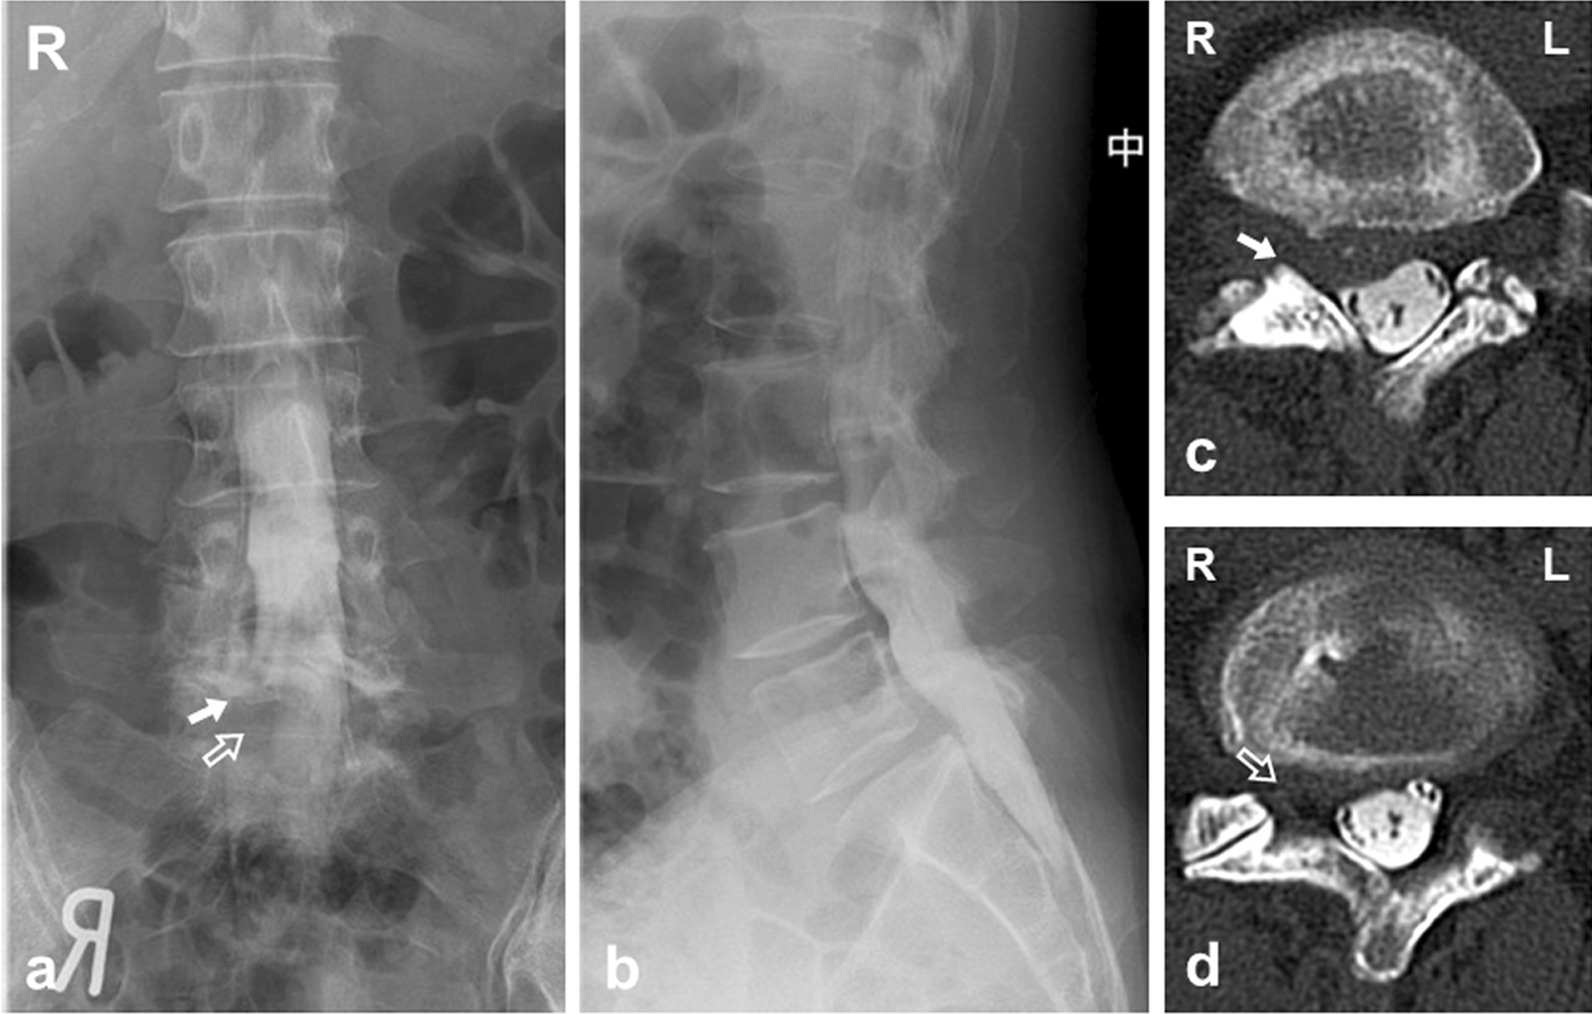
Myelography and CT following myelography (CTM). The defect in the right L5 and S1 nerve roots was shown (white arrow: right L5 root, open arrow: right S1 nerve root). a a-p view. b lateral view. c, d axial view of CTM
